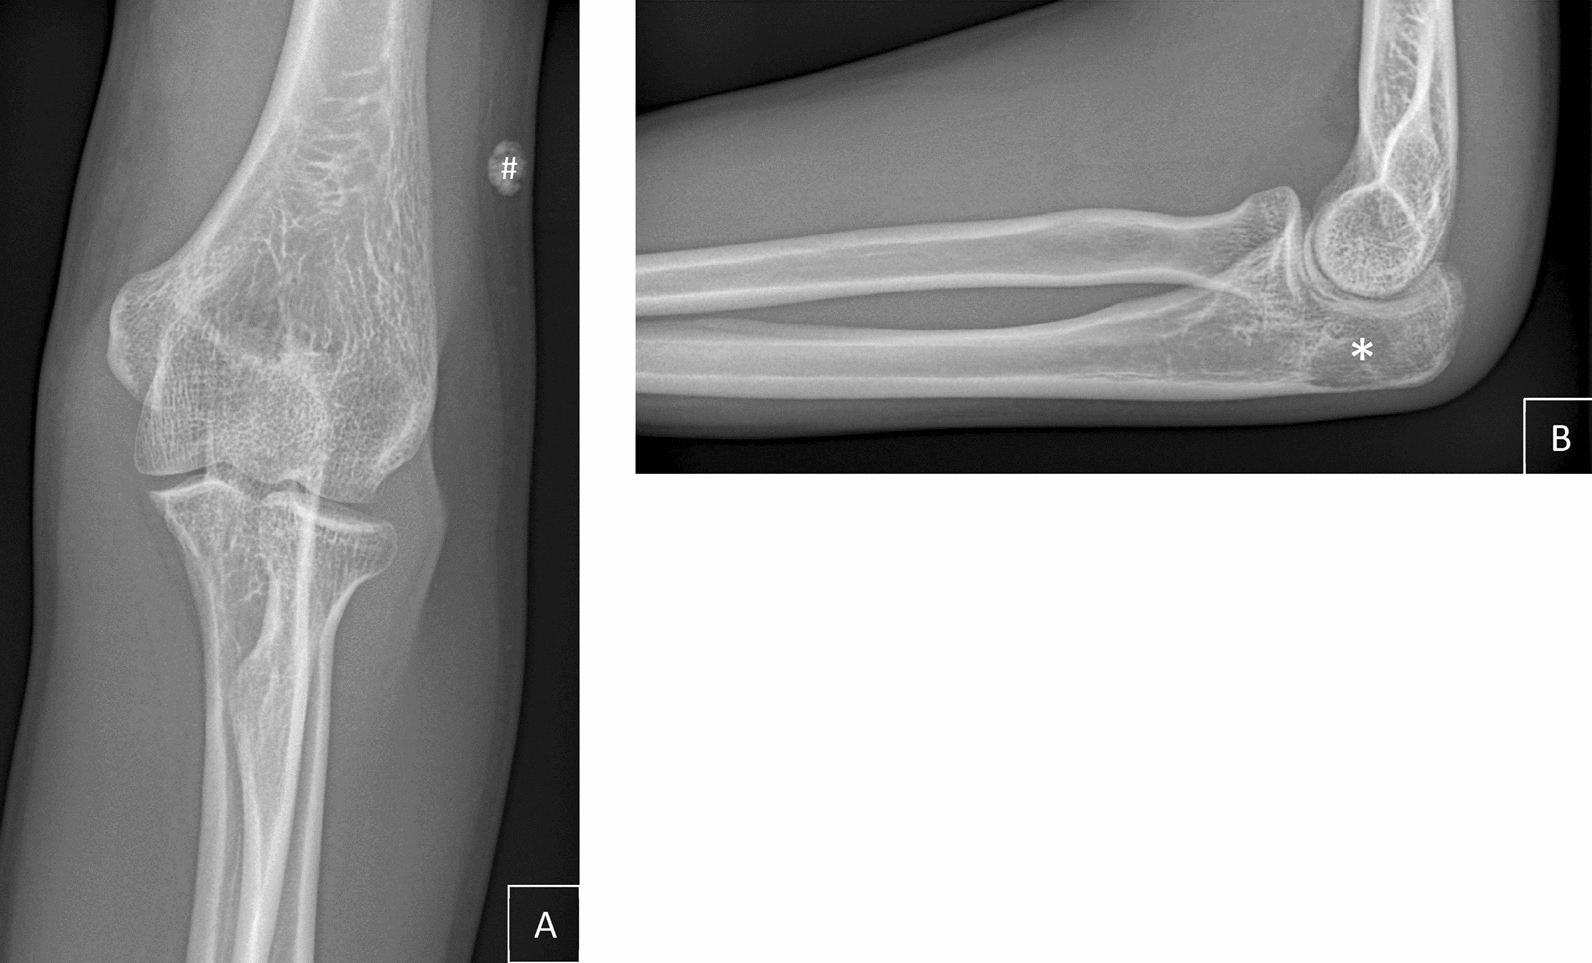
X-ray of the left elbow, taken on 17 April 2020; A anterior–posterior view, # shows unclear soft tissue calcification; B lateral view, *shows potential osteolysis
